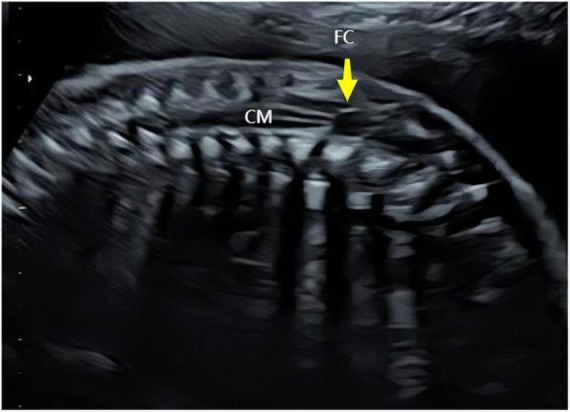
Longitudinal sections of the prenatal ultrasound of the spine. Yellow arrows indicate cysts of the terminal filament (FC) and conus medullaris (CM).
